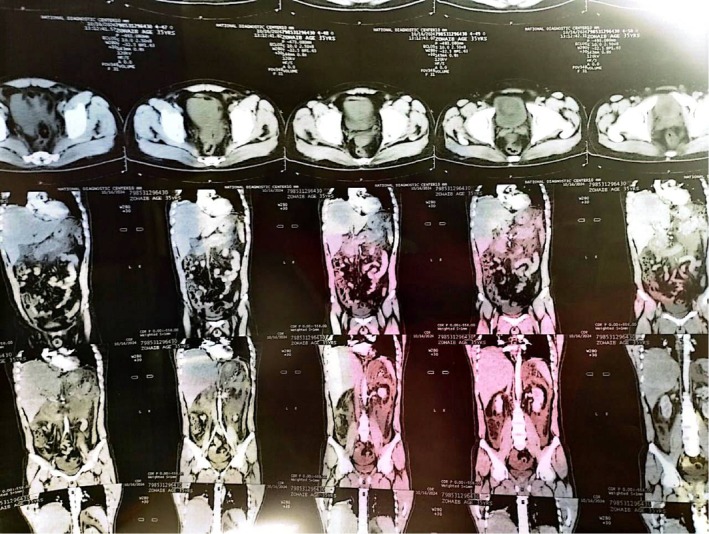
Axial Abdominal CT Scan (Mid Section) Revealing persistent pancreatic inflammation and the beginning of fluid extension into the paracolic gutters.
